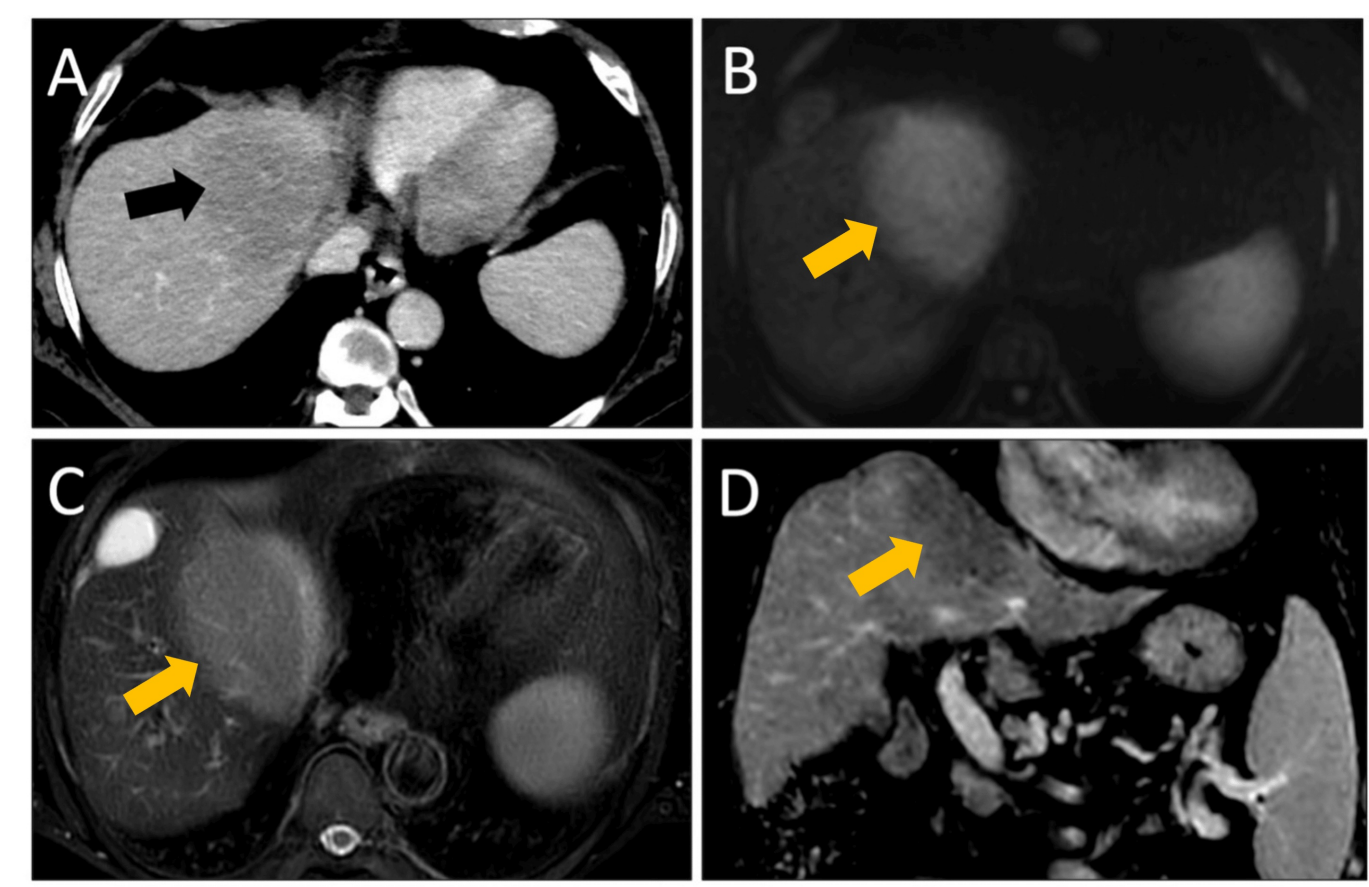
A) Axial CT scan with contrast of the upper abdomen with an ill-defined hypoenhancing subdiaphragmatic lesion (black arrow in A). B) Restricted diffusion on MRI diffusion-weighted imaging within the same lesion (yellow arrow in B). C) Axial T2-weighted MRI with a hyperintense lesion compared to background liver (yellow arrow in C). D) Coronal post-contrast T1-weighted imaging demonstrating subdiaphragmatic location of the hypoenhancing lesion (yellow arrow in D).
